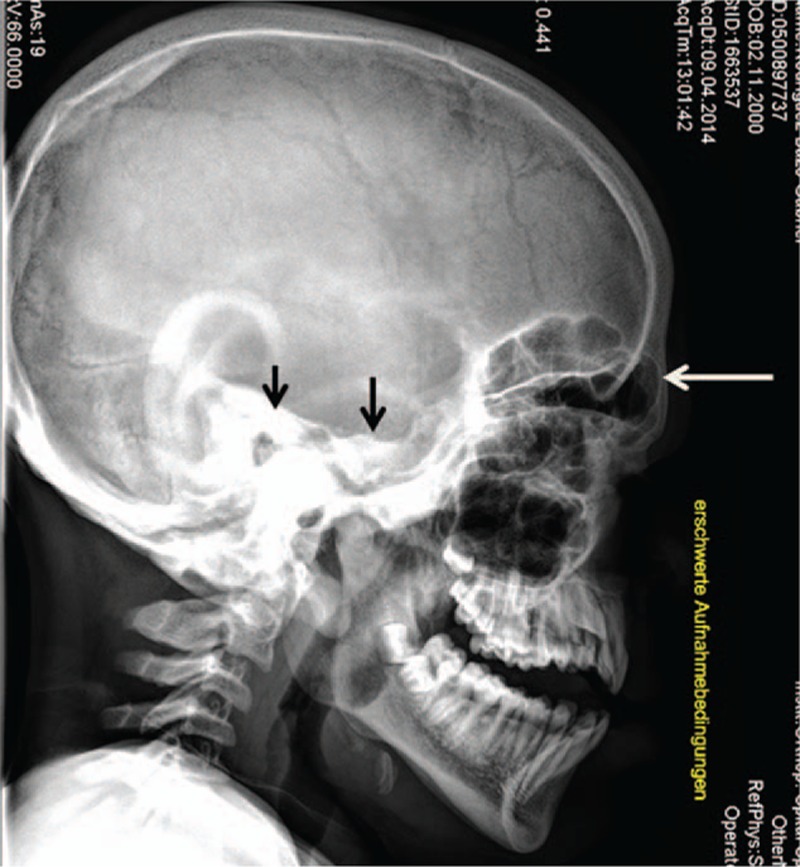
Lateral skull radiograph subject (IV,10) showed thickened sclerosed calvaria, large cella turcica, and hyperostosis of the skull base (arrows).
